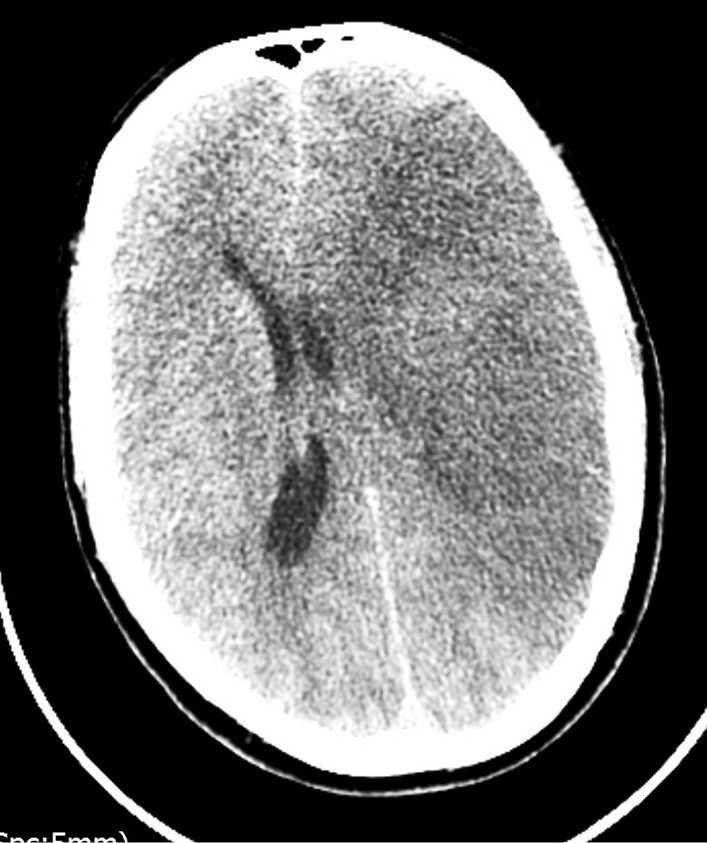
Computed tomography Brain showing L-sided oedema with mass effect
